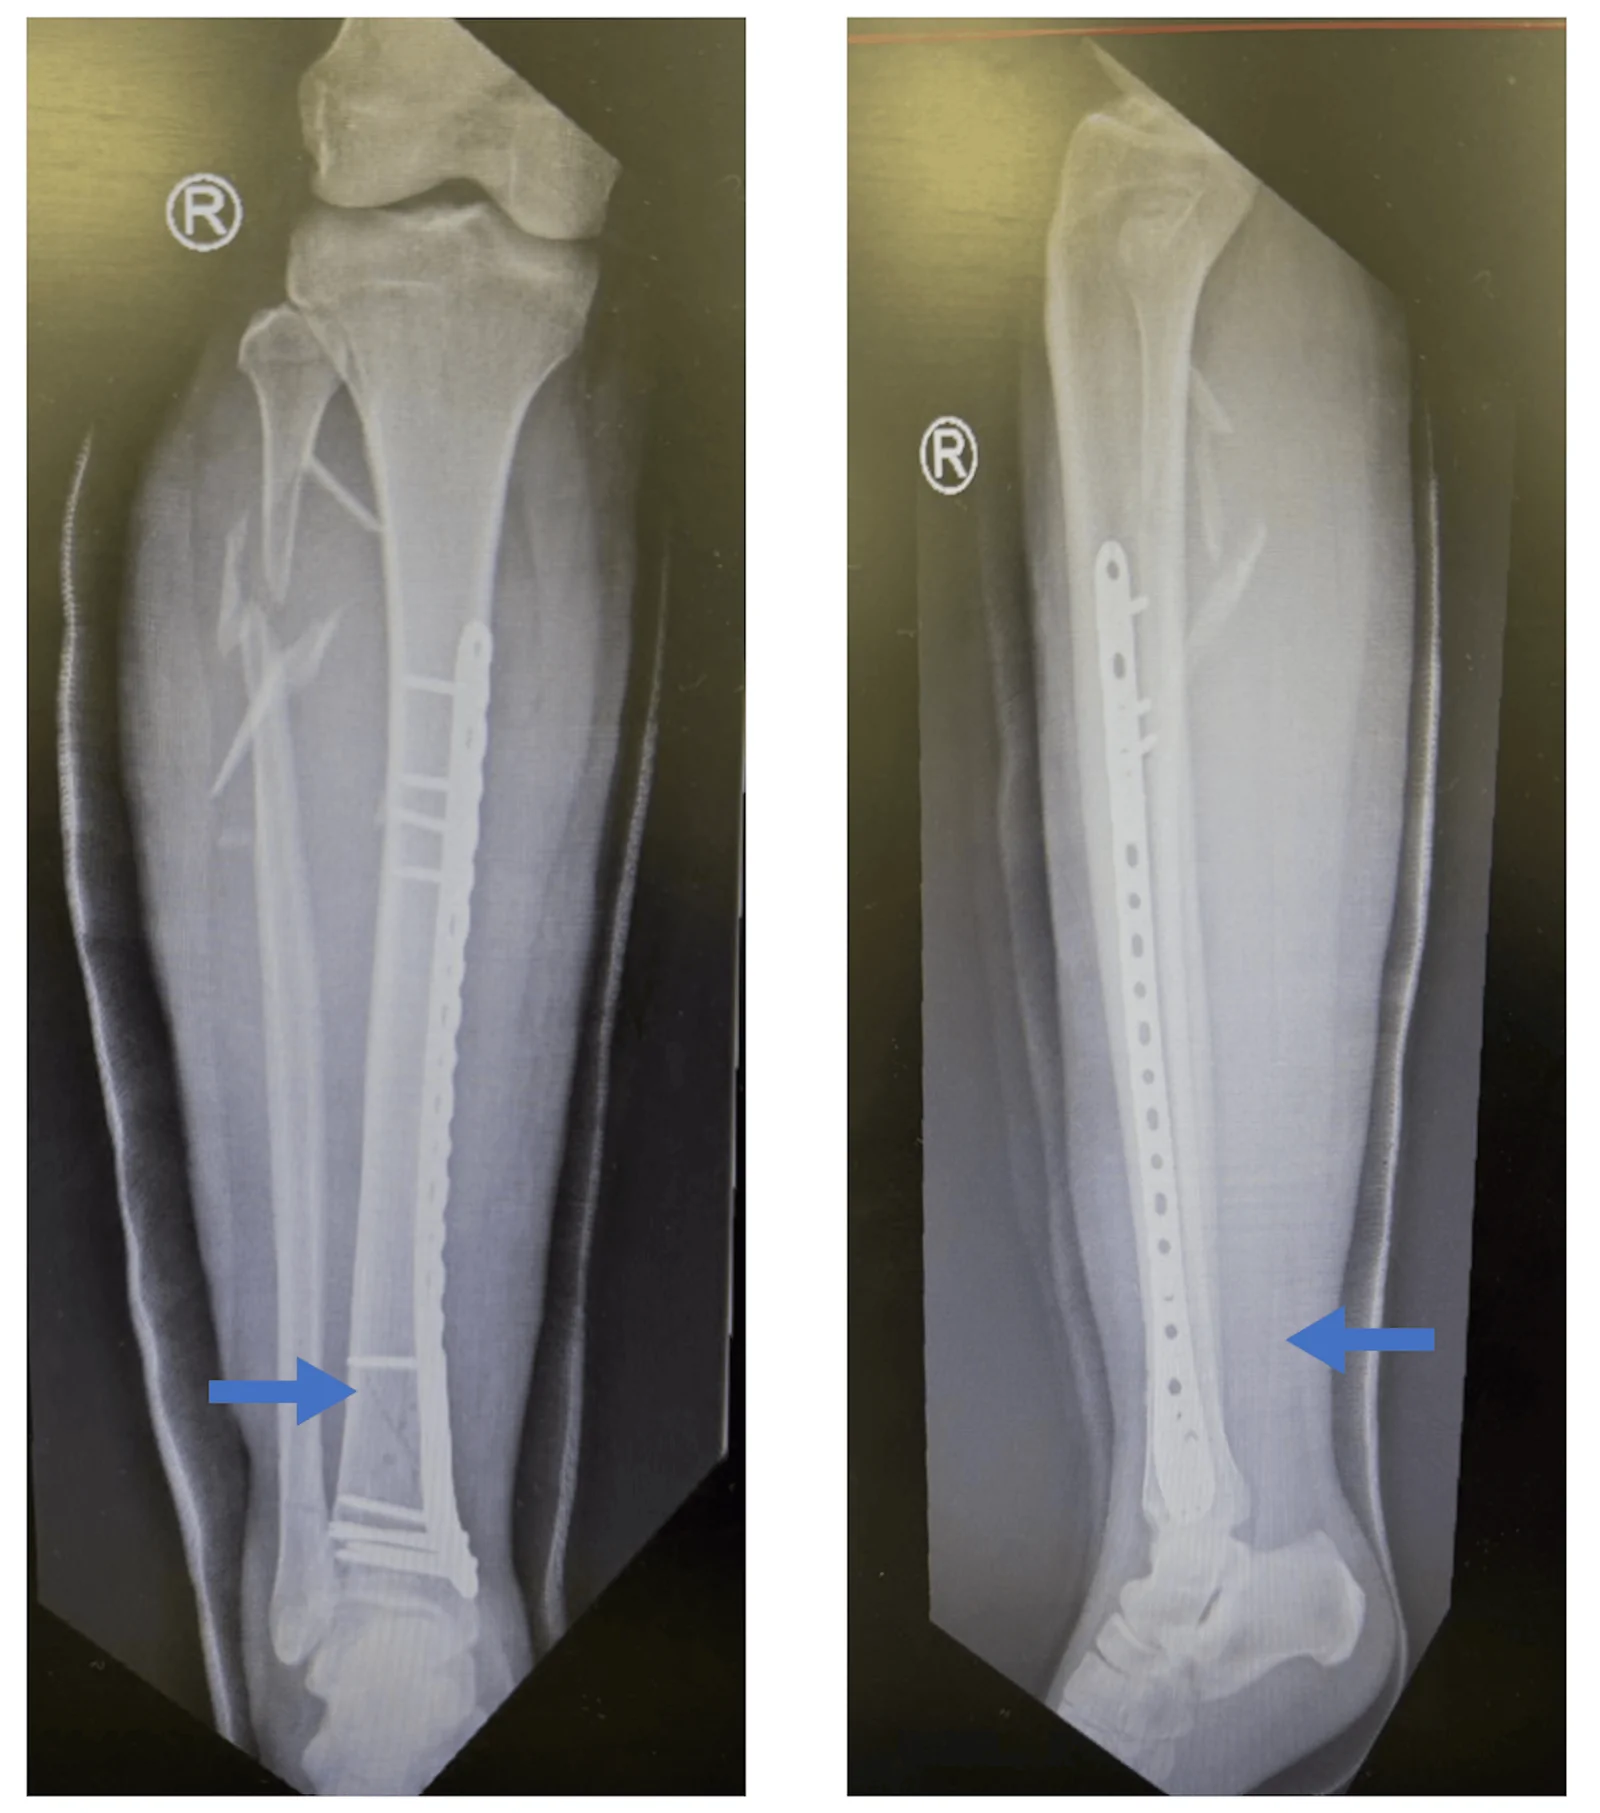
Anteroposterior and lateral postoperative radiographs showing a distal tibia fracture fixed with a locking plate (blue arrow).
